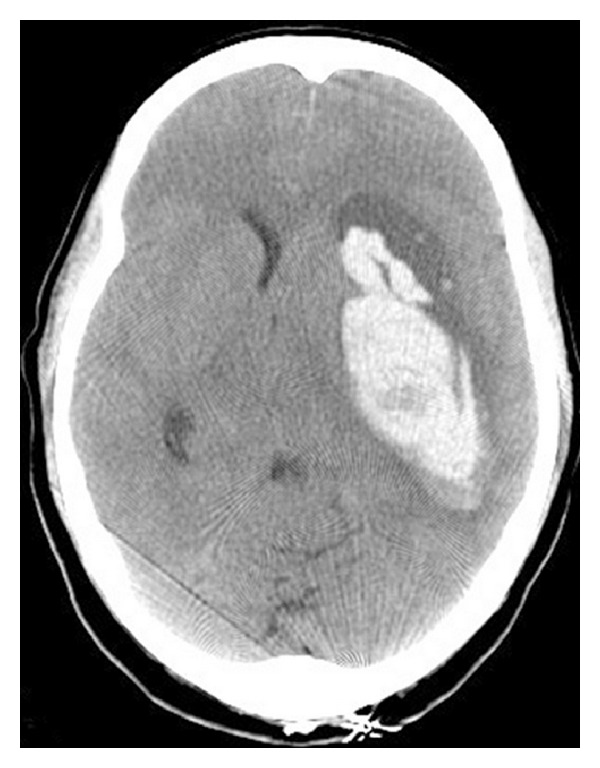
Cranial CT without contrast demonstrating a 9 × 4 × 6.6 cm left deep intraparenchymal hemorrhage without any associated SAH, with 1 cm midline shift.
